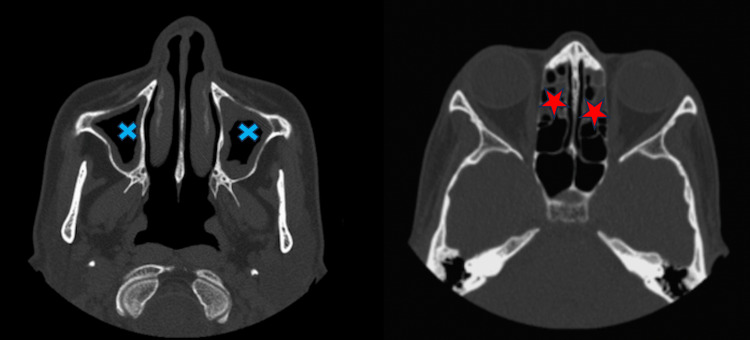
Axial CT images showing maxillary sinus (crosses) and anterior ethmoidal sinus opacification (stars) CT: computed tomography
